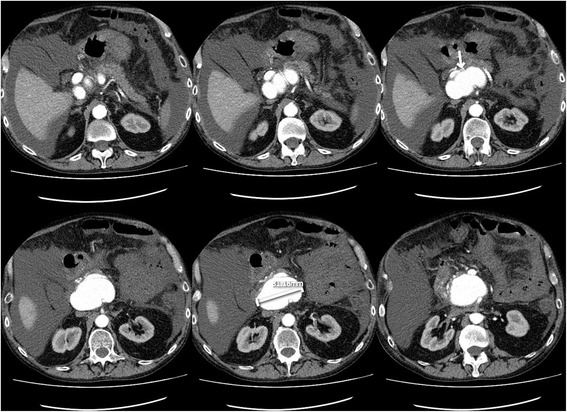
Preoperative computed tomography angiography showing a large pseudoaneurysm between the superior mesenteric artery and coeliac trunk with a diameter of 81 mm. The *arrow* points to a portal fistulization
